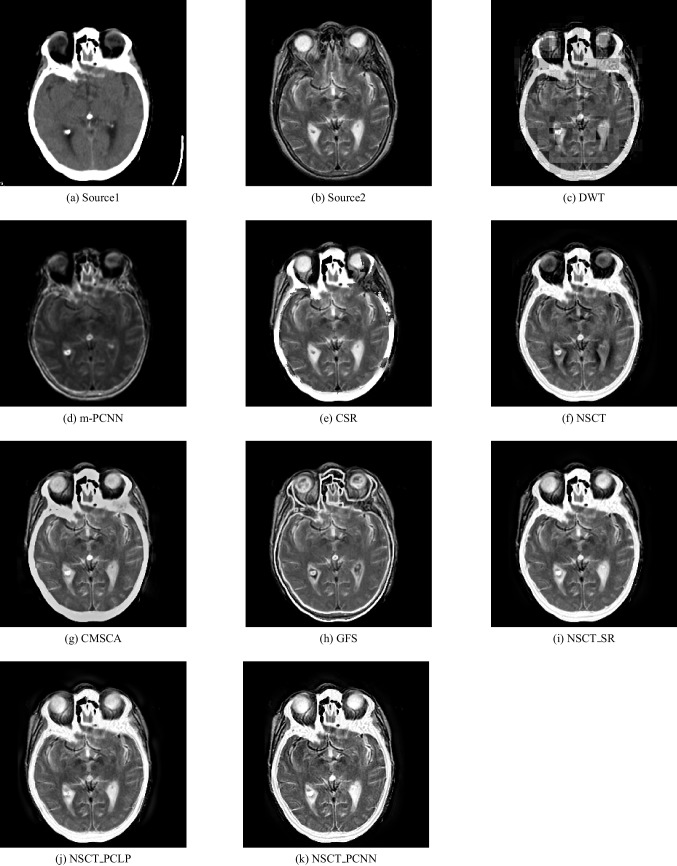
Fusion result of (a) CT and (b) MR-PD using (c) DWT method, (d) m-PCNN method, (e) CSR method, (f) NSCT method, (g) CSMCA method, (h) GFS method, (i) NSCT-SR method, (j) NSCT-PCLP method, and the proposed method (k) NSCT-PCNN
